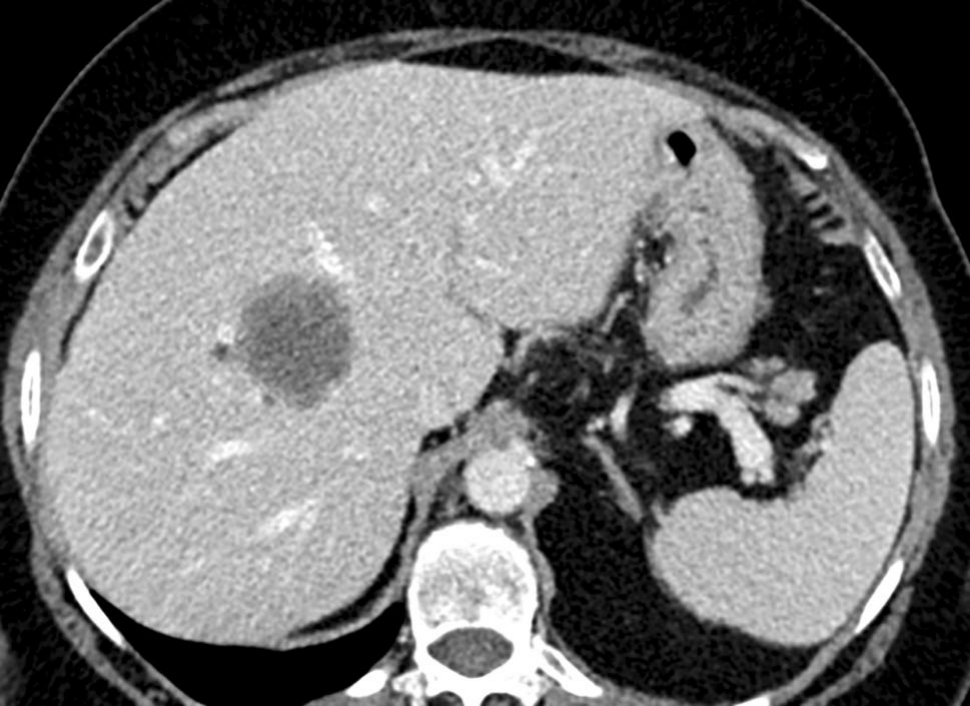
Portal phase of a Computed Tomography scan representing the absence of portal rim enhancement around a liver metastases from colorectal cancer
